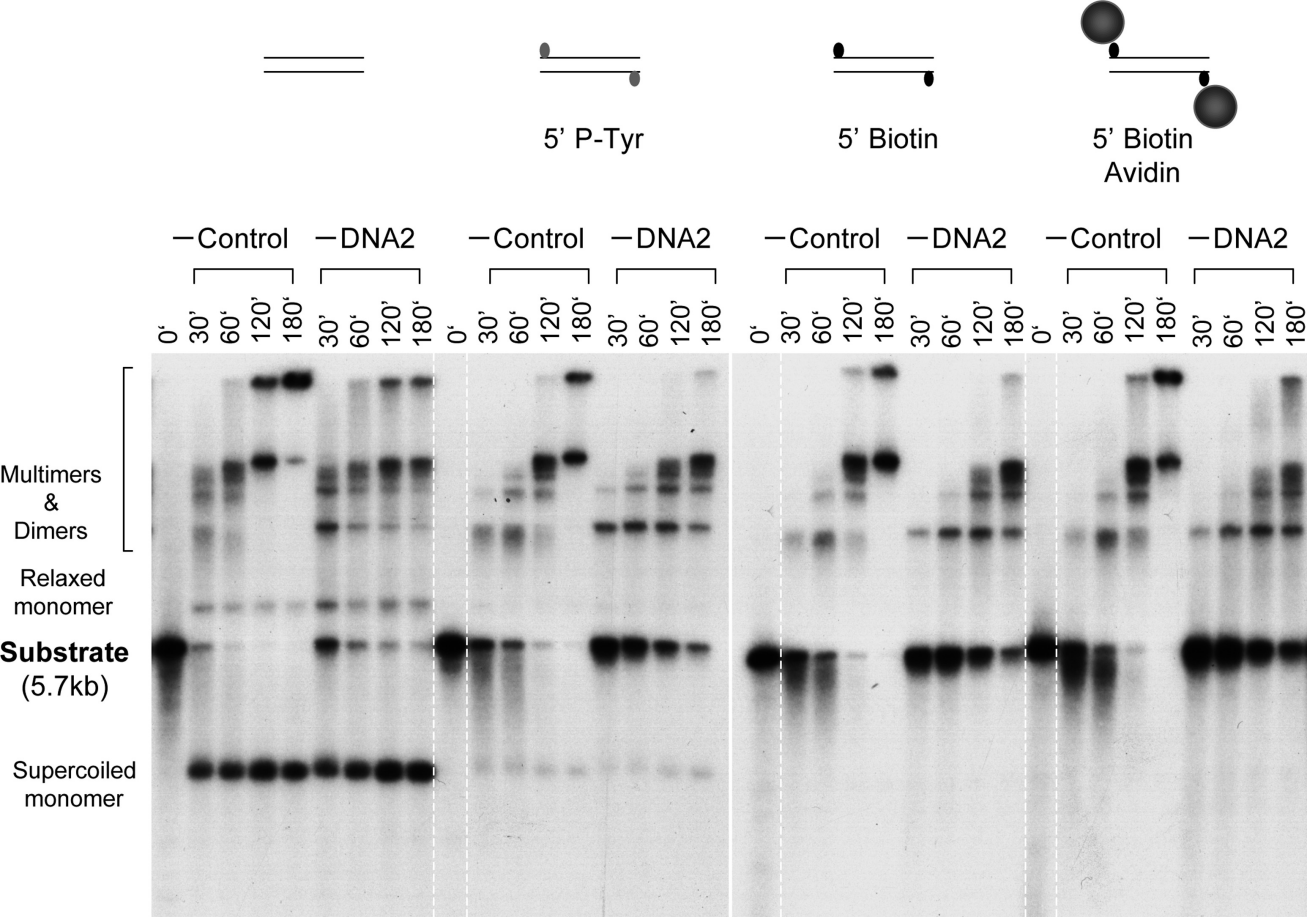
Depletion of DNA2 inhibits resection of DNA with 5′ adducts. (A) DNA substrates bearing different types of ends were incubated at 1.5 ng/μl with the control or DNA2-depleted extracts. Samples were treated with SDS-EDTA-Proteinase K, and separated on an 1% TAE-agarose gel. The gel was dried and exposed to X-ray film.
